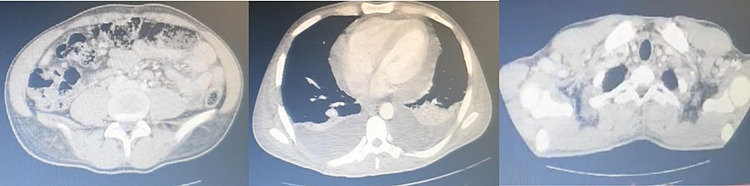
Non-contrast chest, abdomen, and pelvis CT showing bilateral pleural effusion and multiple lymph nodes.
